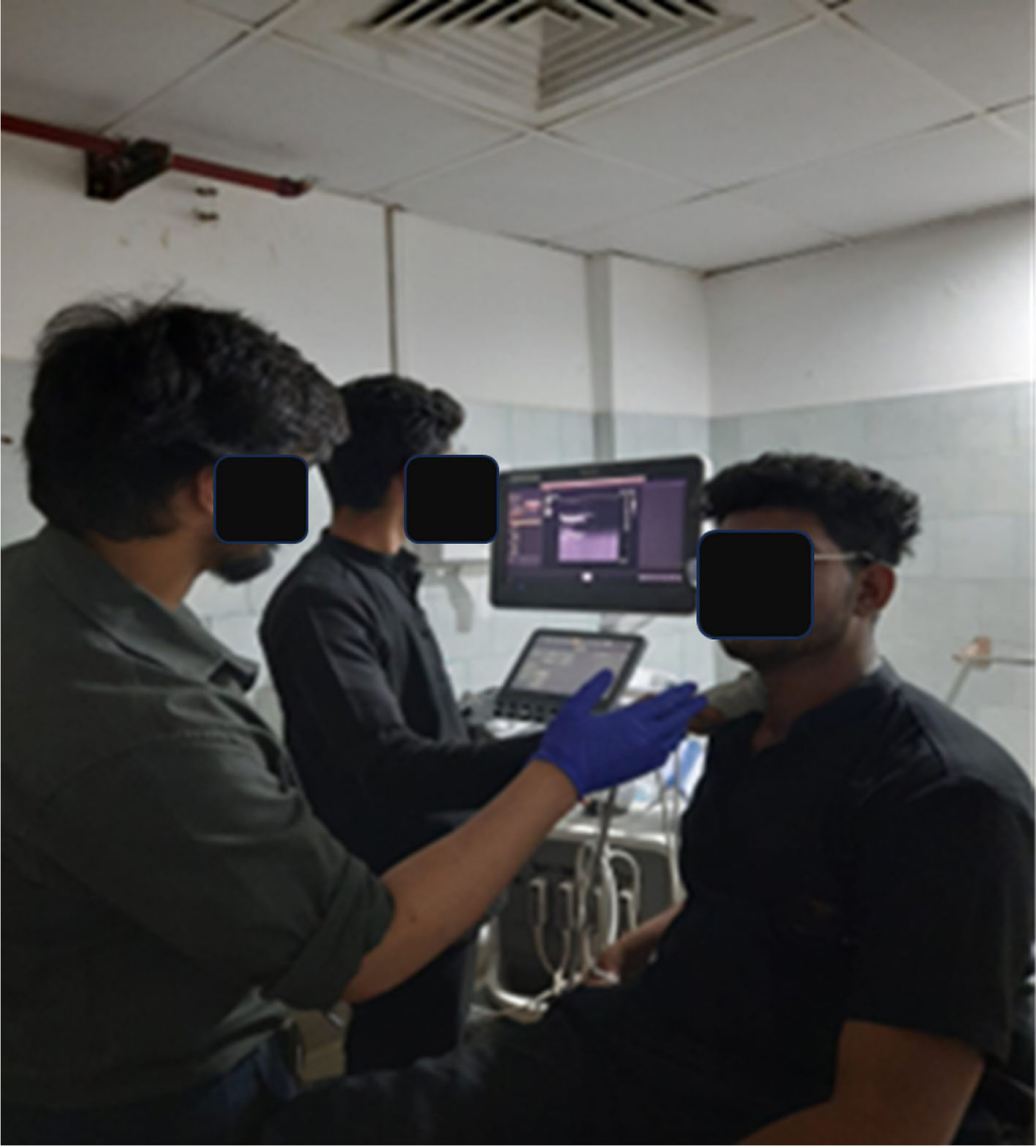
CCA measurements being taken using ultrasound.
